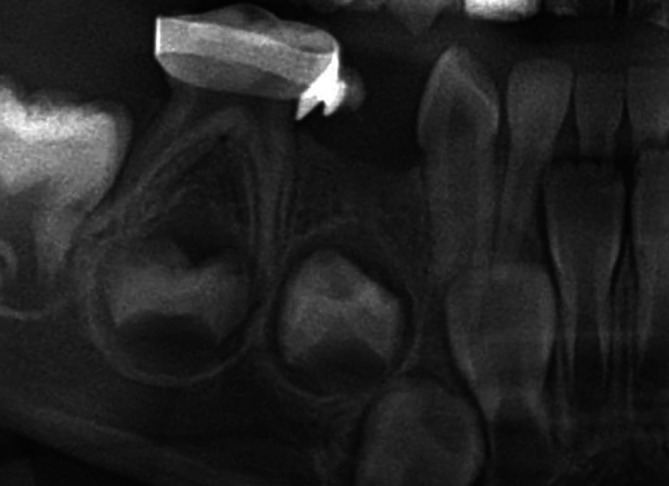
Radiographic follow‐up at 12 months showing preservation of the extraction space and normal development of the permanent successor.
